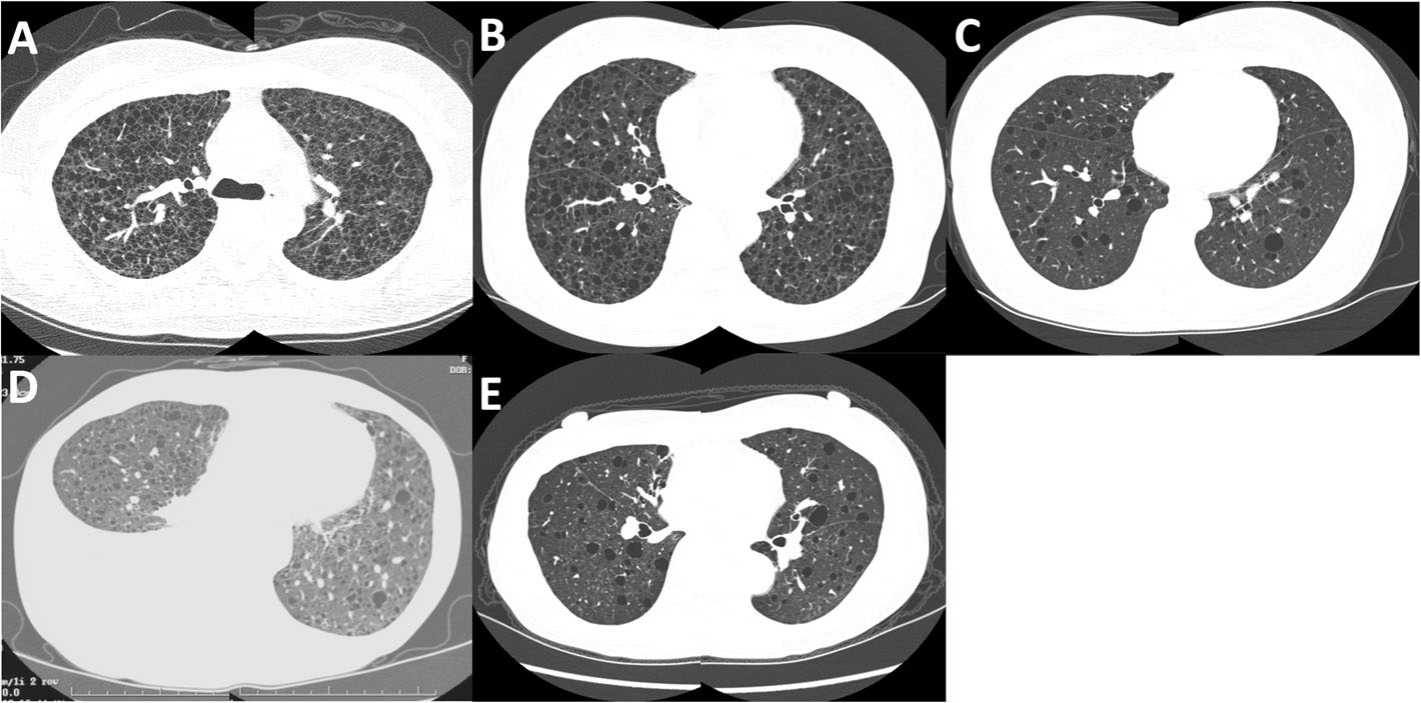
Chest CT findings in five patients with lymphangioleiomyomatosis (LAM) and comorbid connective tissue diseases. All five patients exhibited multiple, diffuse, thin-walled cystic lesions. a Patient 1: A 38-year-old women with LAM, Sjögren’s syndrome, and antiphospholipid antibody syndrome. b Patient 2: A 61-year-old patient with LAM and comorbid Sjögren’s syndrome. c Patient 3: A 48-year-old patient with LAM and comorbid Sjögren’s syndrome. d Patient 4: A 44-year-old patient with LAM and comorbid rheumatoid arthritis. The examinations revealed right pleural effusion. e Patient 5: A 49-year-old patient with LAM and comorbid systemic lupus erythematosus
